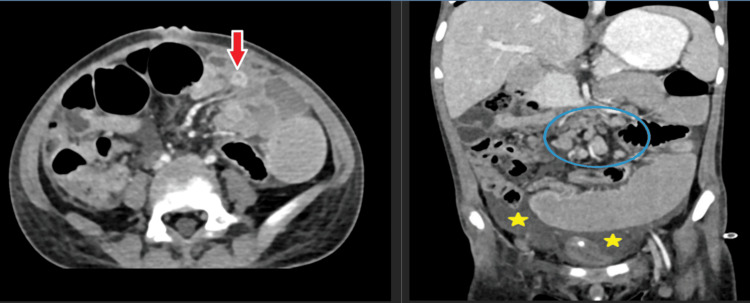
Abdominal tuberculosis with small bowel obstruction (case 1). Contrast-enhanced computed tomography (CECT) abdomen axial section shows multiple dilated small bowel loops due to strictures (red arrows). The coronal section displays enlarged mesenteric lymph nodes (blue circle) and ascites (yellow stars).
